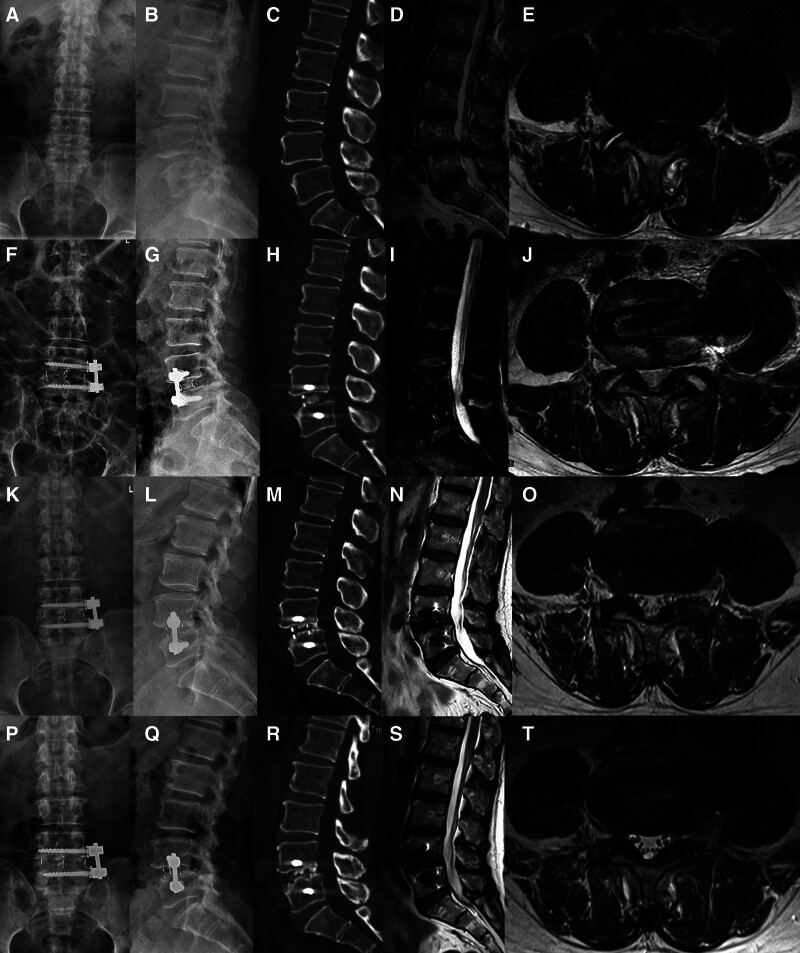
A patient of L4–L5 SLSS underwent OLIF–AF. (A–E) A–P and lateral X-ray, sagittal CT, sagittal and horizontal MRI preoperatively; (F–J) A–P and lateral X-ray, sagittal CT, sagittal and horizontal MRI 1 day postoperatively; (K–O) A–P and lateral X-ray, sagittal CT, sagittal and horizontal MRI 12 months postoperatively; (P–T) A–P and lateral X-ray, sagittal CT, sagittal and horizontal MRI 24 months postoperatively. CSA expanded after OLIF–AF and expanded further during follow-up. Derotation and partial reduction of slippage were accomplished and stayed relatively stable. DH slightly deceased but CS did not occur, no fixation loosening was observed and fusion was accomplished.
